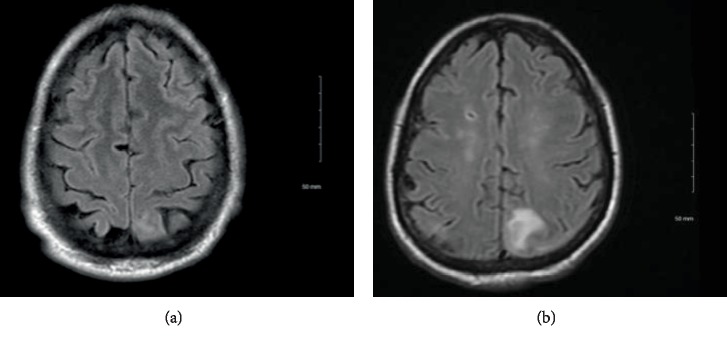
Magnetic resonance imaging of the brain showing progression of leptomeningeal disease. (a) Hyperintensity can be seen in L parasagittal sinus in this T2 Flair at time of diagnosis of LMD. (b) Increased hyperintensity in the same region in this T2 Flair taken 4 months after diagnosis of LMD.
